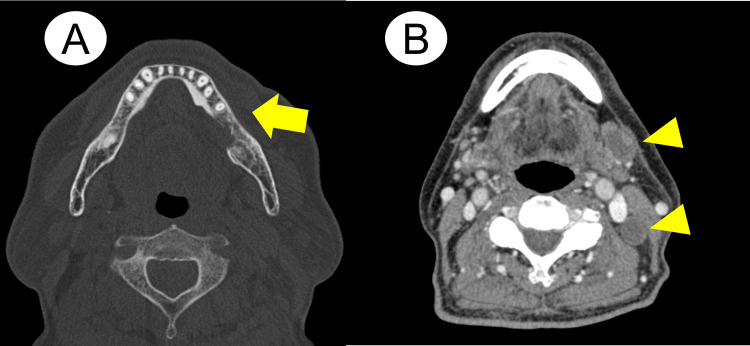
Contrast enhanced CT findings at first appearance A: Bone resorption was detected in the left mandibular alveolar region (arrow); B: Multiple cervical lymph nodes displayed rim-enhancing findings, indicating lymph node metastases (arrowheads)
